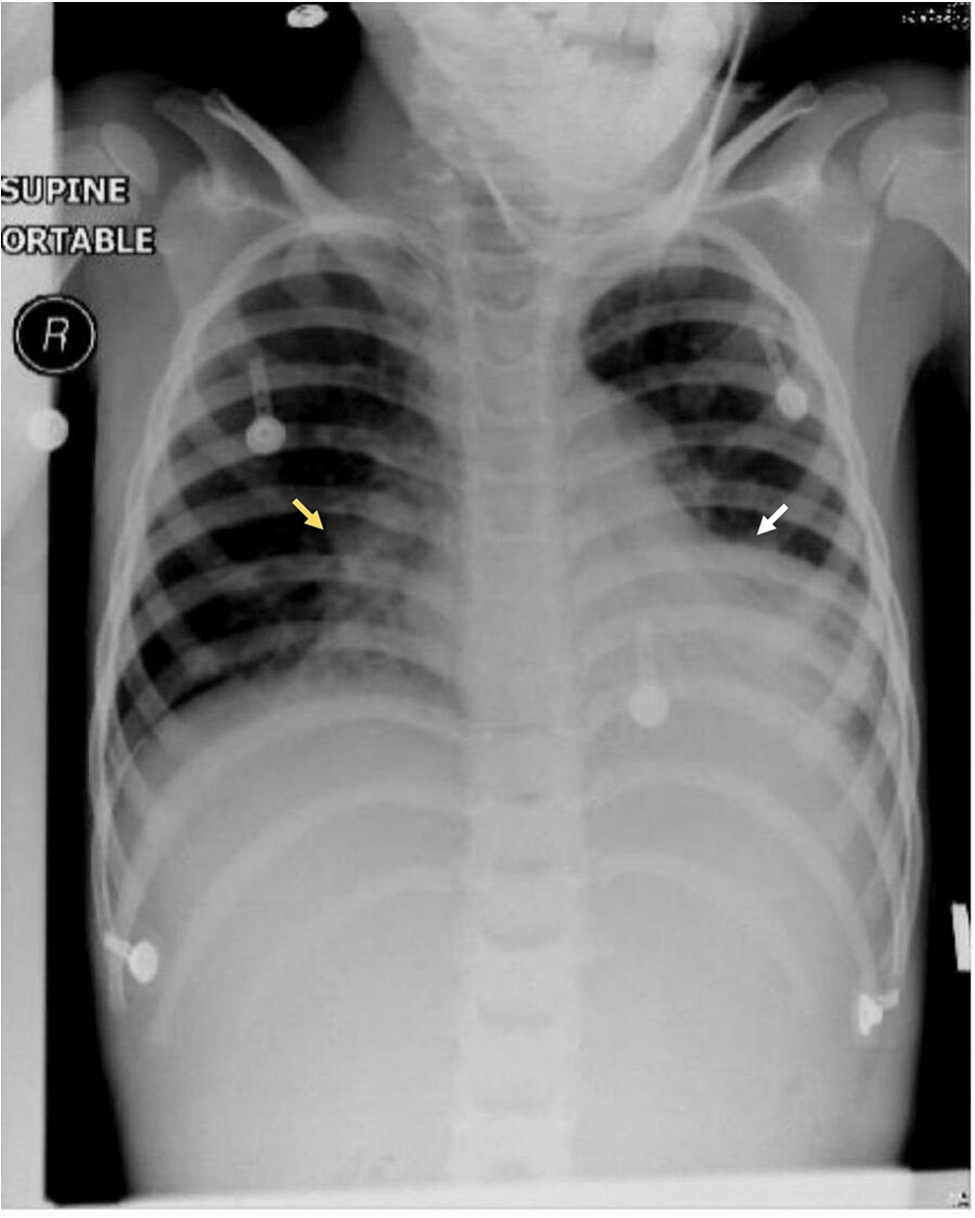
Plain chest x-ray, anteroposterior view, showing opacity in the left lower zone with air bronchogram silhouetting the cardiac border and cardiophrenic border (white arrow) and right-sided peribronchial wall infiltrate (yellow arrow), with compensatory mild hyperinflation
